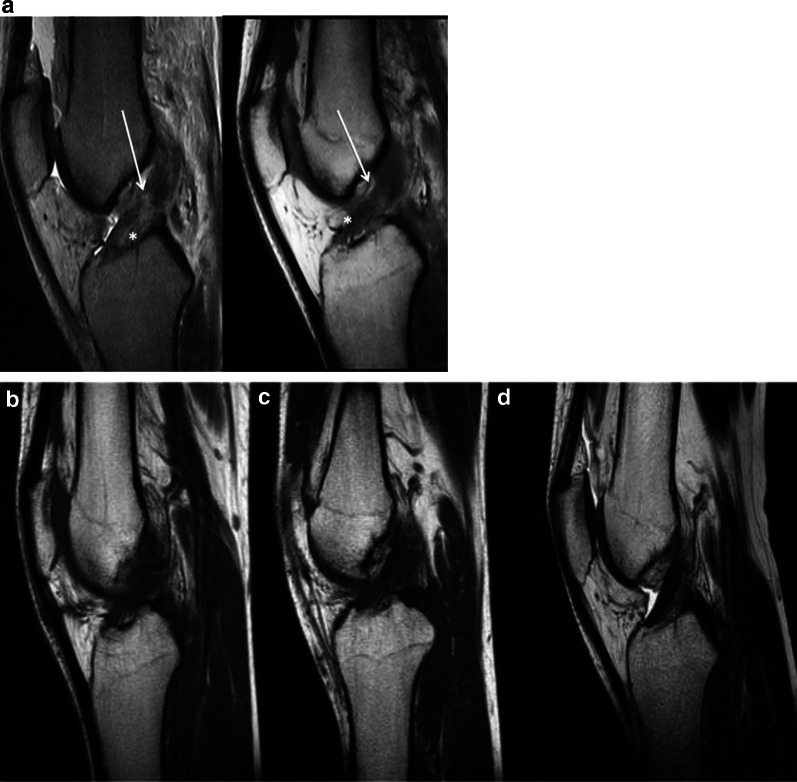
Pre- and postoperative images of patient #5. a Sagittal preoperative MRI T2-TSE (left) and T1-TSE (right). Tear location (arrow): type III; tissue quality (asterisk): good b Sagittal T1-TSE 1 month post-op. Morphology: grade 1 (normal); signal intensity: grade 2 (intermediate) c Sagittal T1-TSE 3 months post-op. Morphology: grade 1 (normal); signal intensity: grade 1 (isointense) d Sagittal T1-TSE 6 months post-op. Morphology: grade 1 (normal); signal intensity: grade 1 (isointense) *TSE* turbo spin-echo
